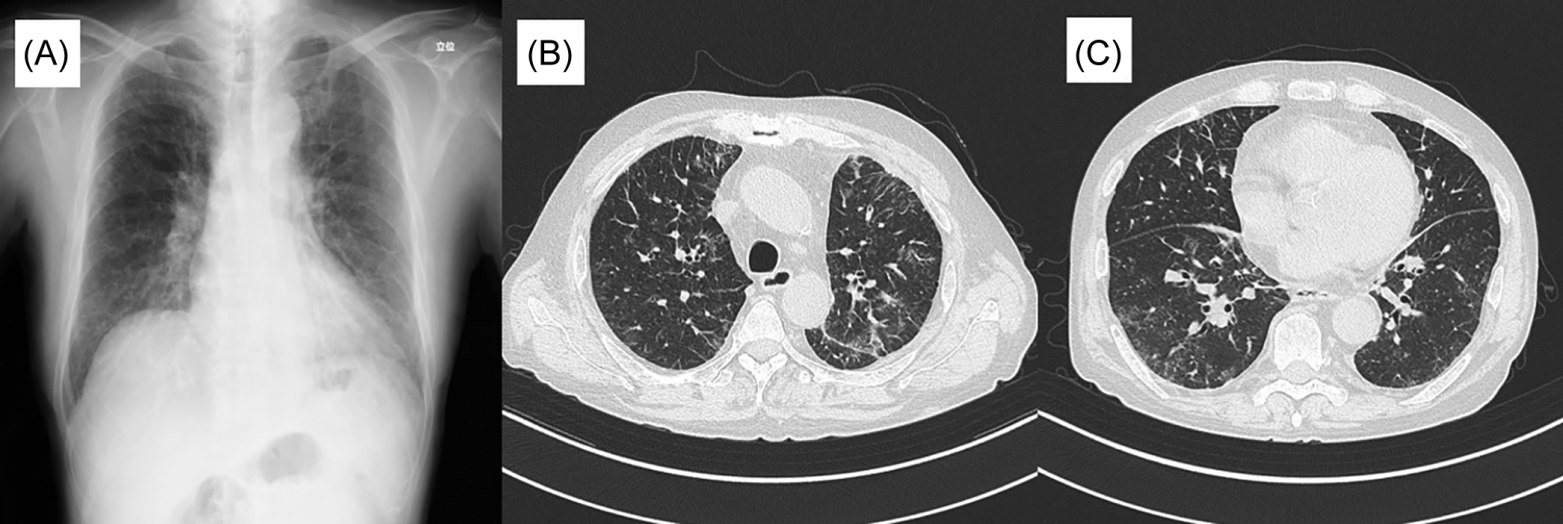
Imaging findings during the initial visit. (A) Chest x‐ray showing bilateral lung hypolucency and reticular opacities. (B and C) Chest computed tomography showing bilateral ground‐glass opacities, hypertrophic interlobular septa, mild mediastinal lymphadenopathy, and slight bilateral pleural effusions.
